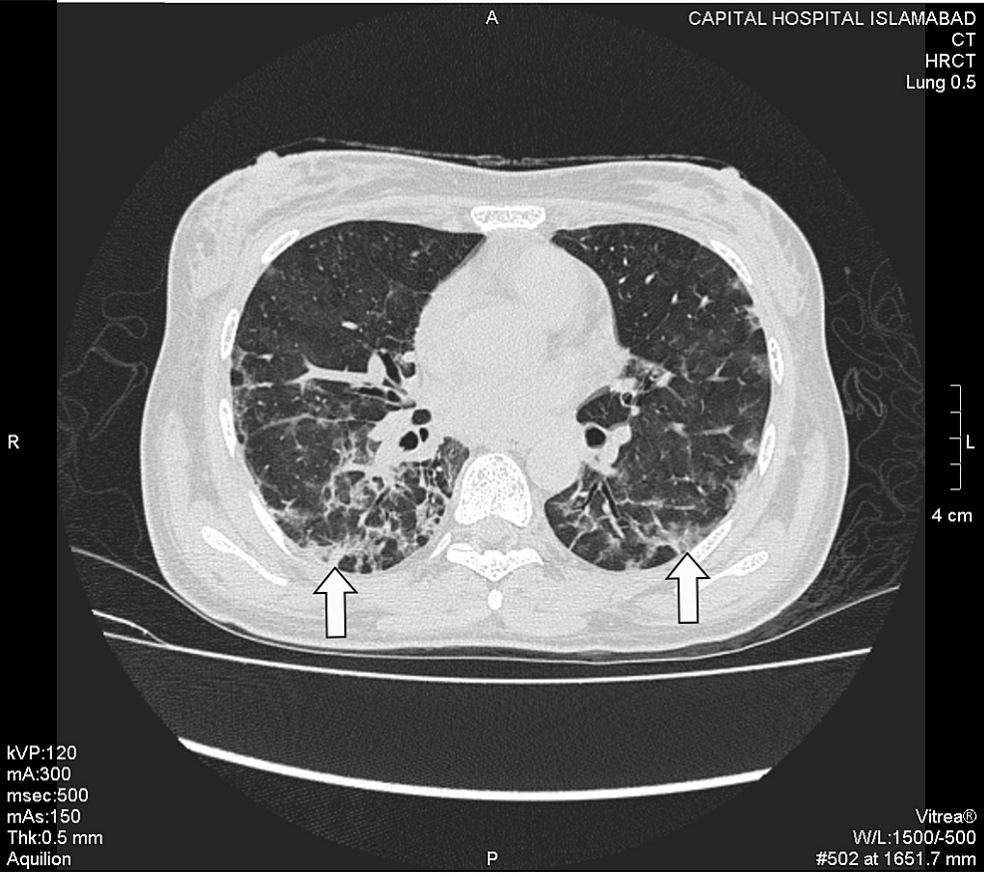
A female patient with severe symptoms. HRCT shows multiple ground-glass opacities mixed with consolidation in bilateral lower lobes (white arrows). HRCT: High-resolution computed tomography.
